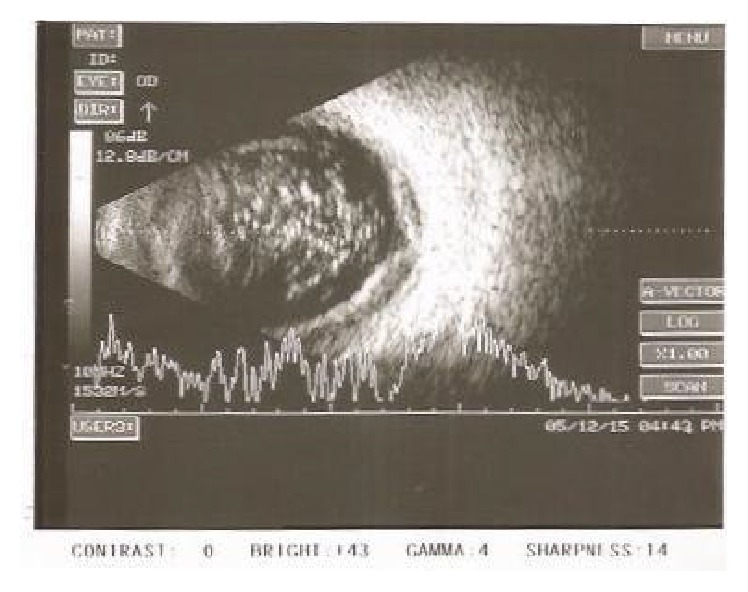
Left eye B-scan of Patient 2: ultrasonography shows asteroid hyalosis and shallow choroidal detachment.
